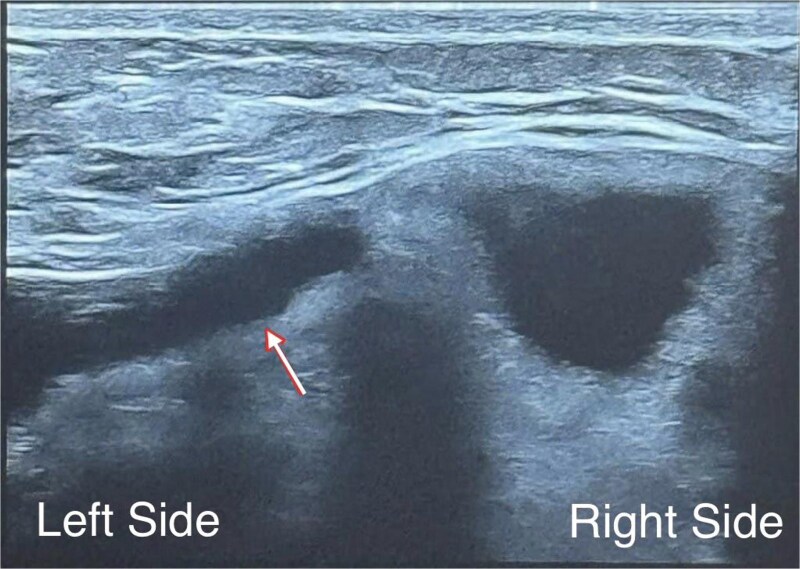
Diagnosis based on breast ultrasonography at 32 + 5 weeks’ gestation: We observed a multiloculated internal appearance (arrow), interpreted as intracapsular rupture, with no peri-implant seroma, extracapsular silicone, or regional lymphadenopathy; the right implant appeared intact.
